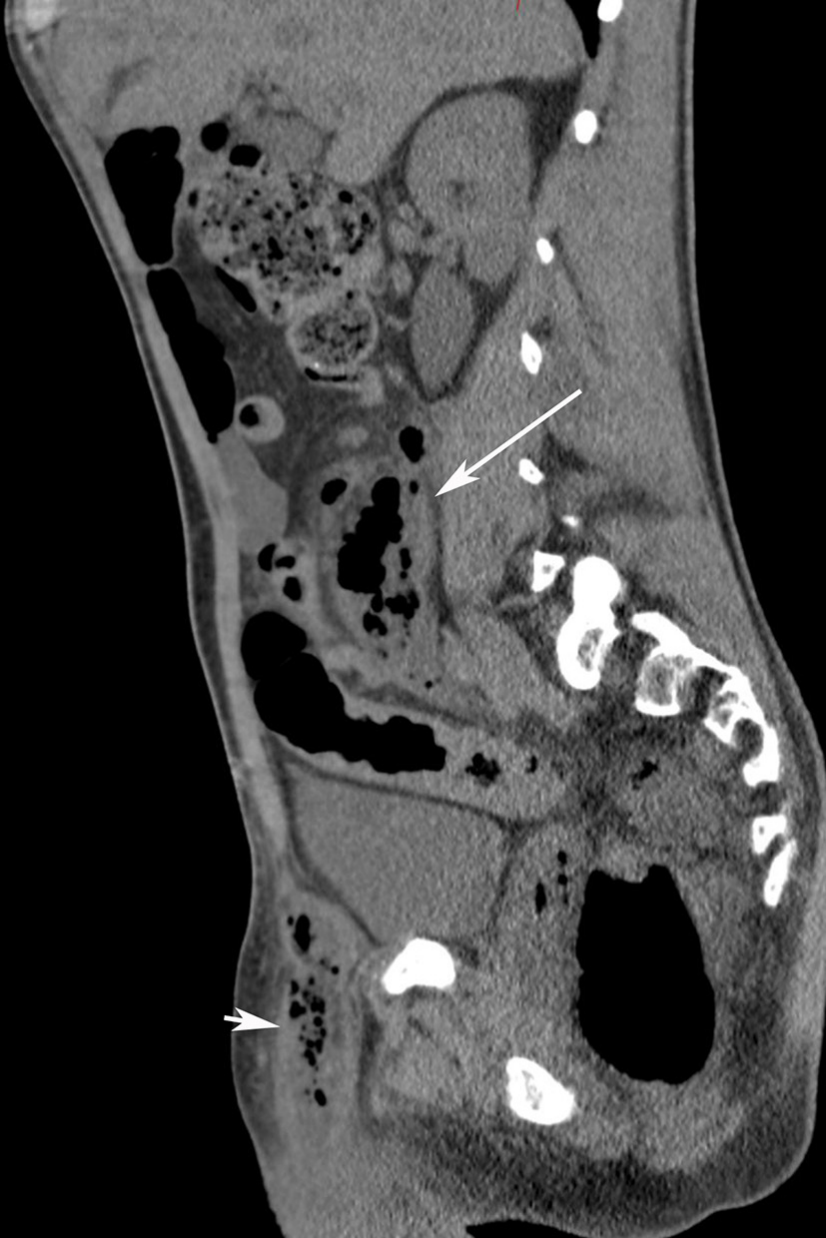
Sagittal venous phase contrast-enhanced CT scan showing the retroperitoneal abscess tracking up from the pelvis (long arrow) and an inguinal abscess (short arrow).
